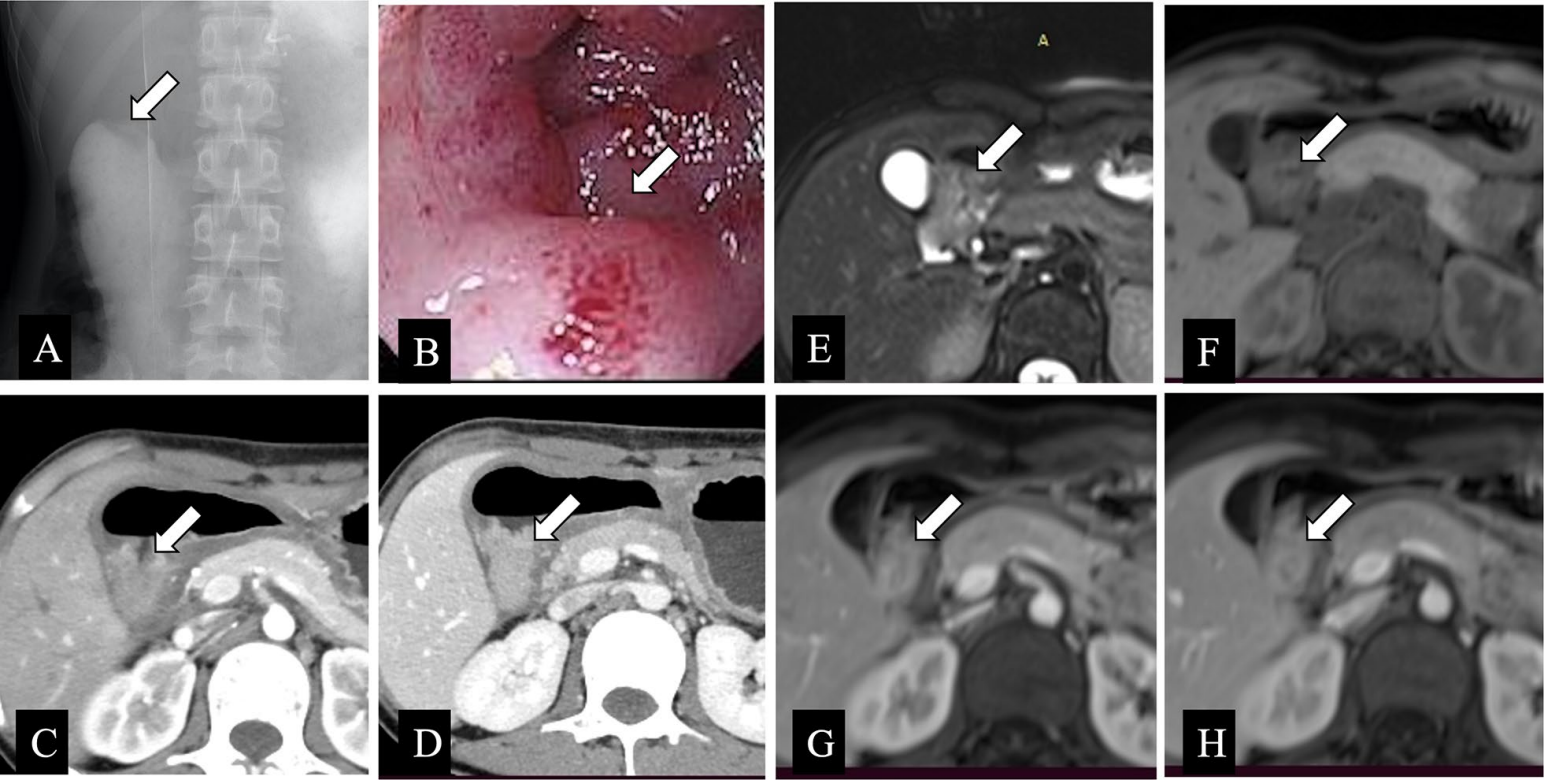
A 15-year-old female presented with abdominal pain and distension underwent various imaging examinations. a Single spot image of the upper gastrointestinal tract on a barium fluoroscopic study demonstrated an intraluminal filling defect within the duodenum with a central indentation (white arrow) consistent with heterotopic pancreas. b Endoscopy showed a subepithelial lesion (white arrow) along the first portion of the duodenum with surrounding duodenitis. c–d Contrast-enhanced axial CT images revealed a hyper-enhanced lesion (white arrows) with ill-defined margin and thickening of the surrounding duodenal wall. e–h Non-contrast axial T2-weighted (e), pre-contrast T1-weighted (f), post-contrast arterial T1-weighted (g), and post-contrast venous T1-weighted images (h) showed a lesion within the first portion of the duodenum (white arrows) demonstrating T2-weighted slightly hyperintense signal, pre-contrast T1-weighted slightly hypointense signal, and post-contrast T1-weighted isointense like those of the normal pancreas
